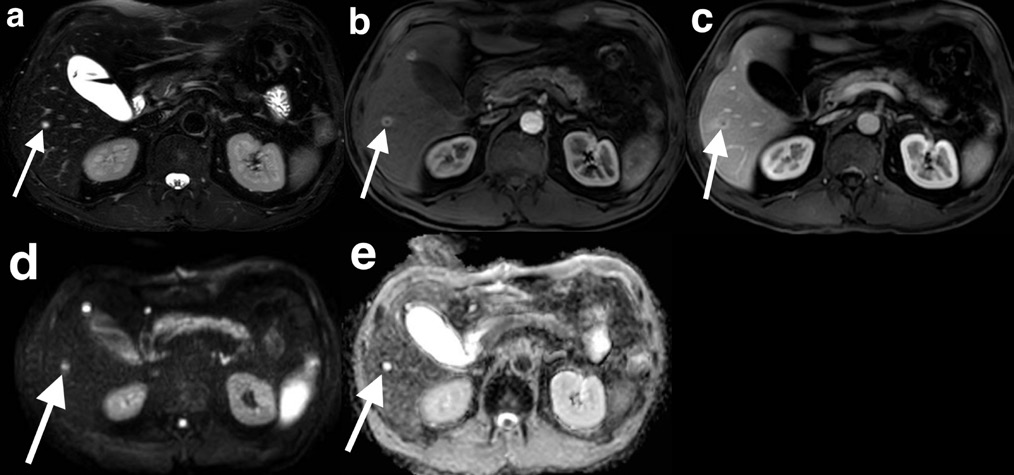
Gadoxetic acid-enhanced MRI showing diffusion weighted imaging appearances. A *T*
